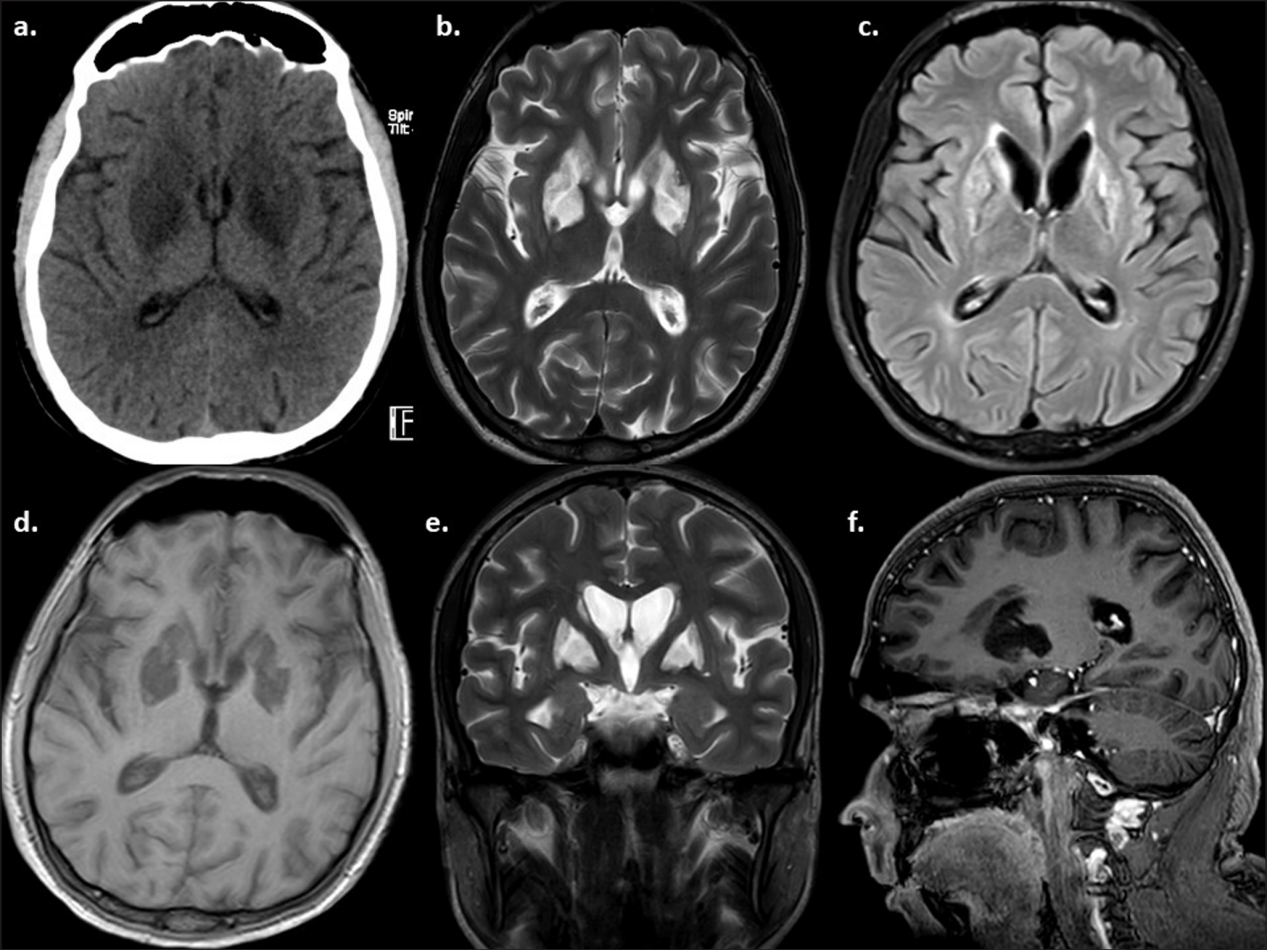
CT brain axial (a) showing hypo density in bilateral basal ganglia and MRI findings (b)-T2 Axial, (c)-FLAIR Axial, (d)-T1 Axial, (e)-T2 coronal sequences showing bilateral basal ganglia involvement. (f)-T1 coronal at the level of basal ganglia without any contrast enhancement.
